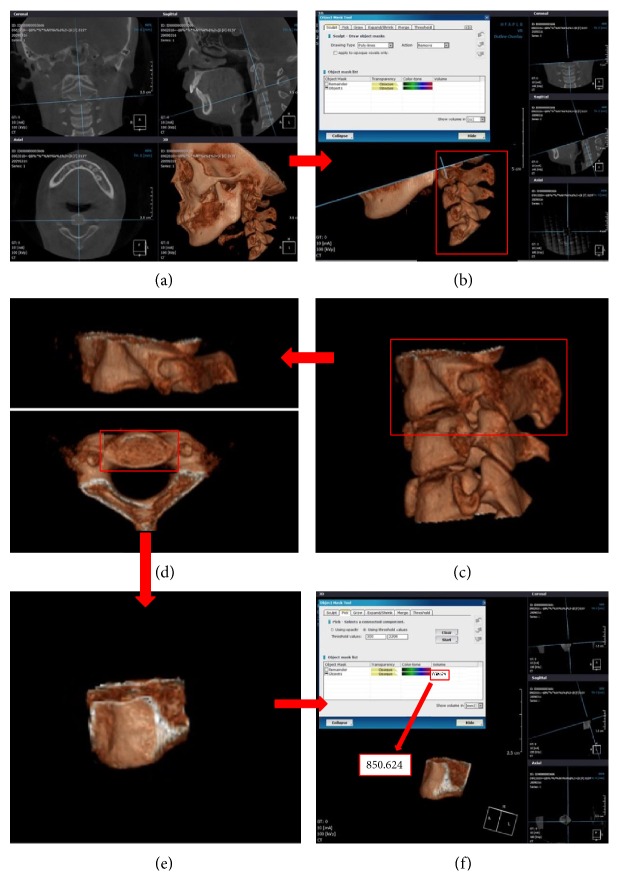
Volume measurement process. CBCT image isolated from each cervical vertebra (C2–C4). (a) The initial full field image. (b)–(e) “Remove” function on manual segment tool was in progress. (f) Finally, the volume of each cervical vertebra was obtained.
